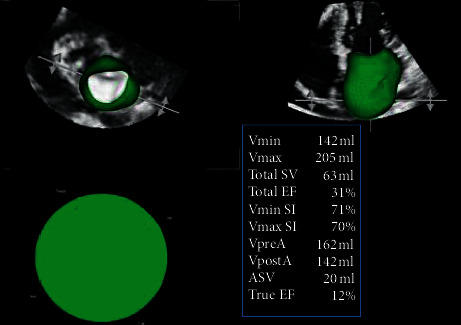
Left atrial quantitative analysis using 3D transthoracic echocardiography in a patient with dilated cardiomyopathy and functional mitral regurgitation.
